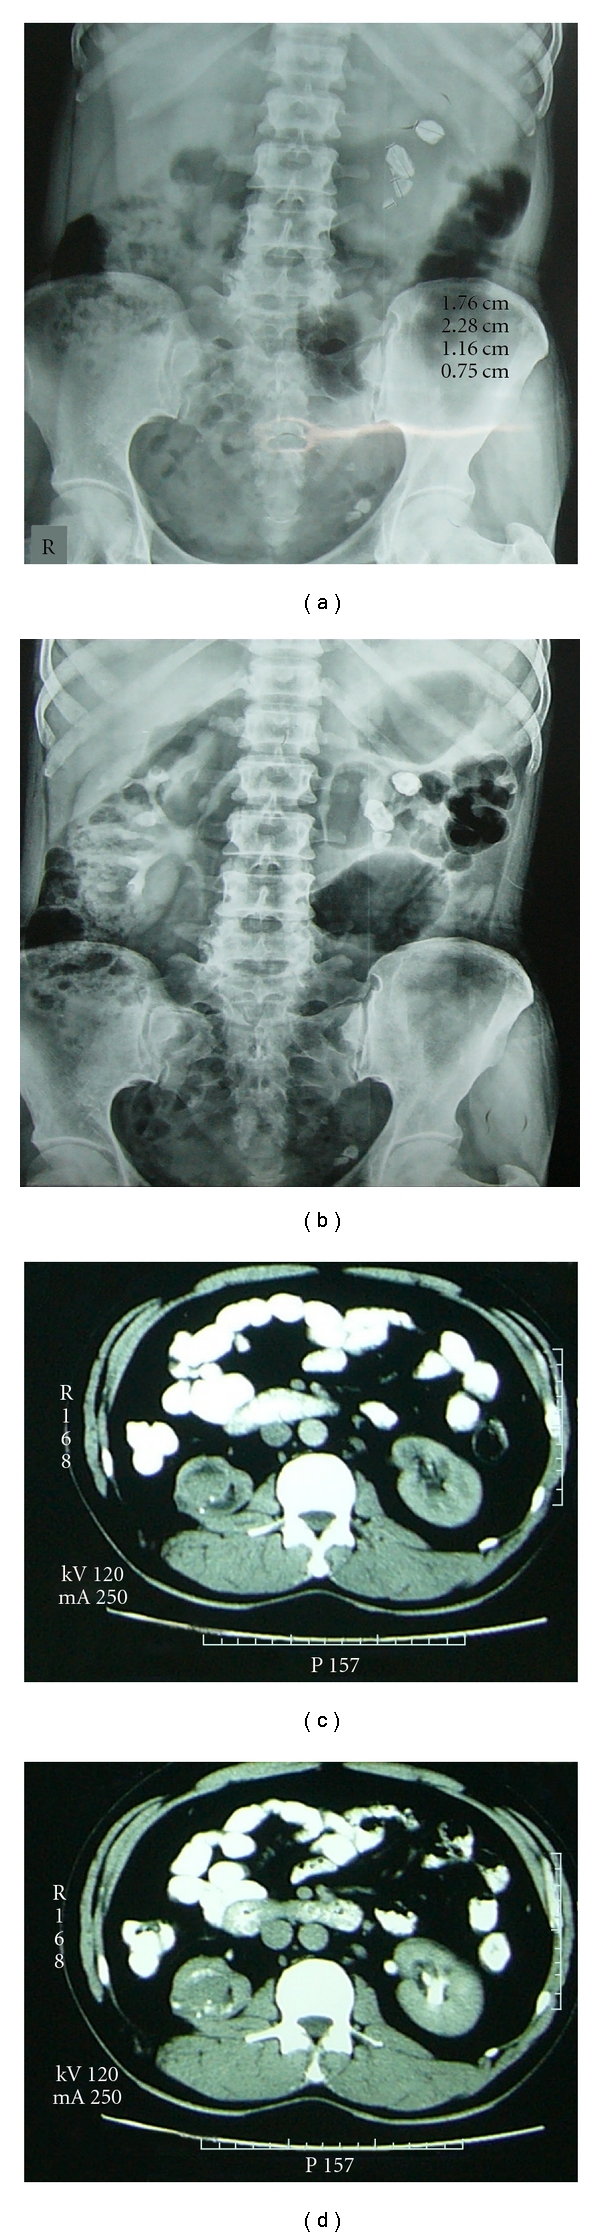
Radiological images. (a) Plain X-ray (KUB) of Case 3 showing multiple radio-opaque shadows in the left kidney; (b) IVP skiagram of the same case at 15 min showing normal excretion of dye in the right kidney while there is complete absence of excretion in the left kidney. Dye excretion was not seen in the left kidney even up to 24 hours. Multiple renal stones can be seen in the left kidney similar to (a); (c) CT scan image of Case 4 showing small and irregular hydronephrotic right kidney with a nodule in the region of pelvis; (d) CECT image of Case 4 showing focal contrast enhancement in the nodule in pelvis.
